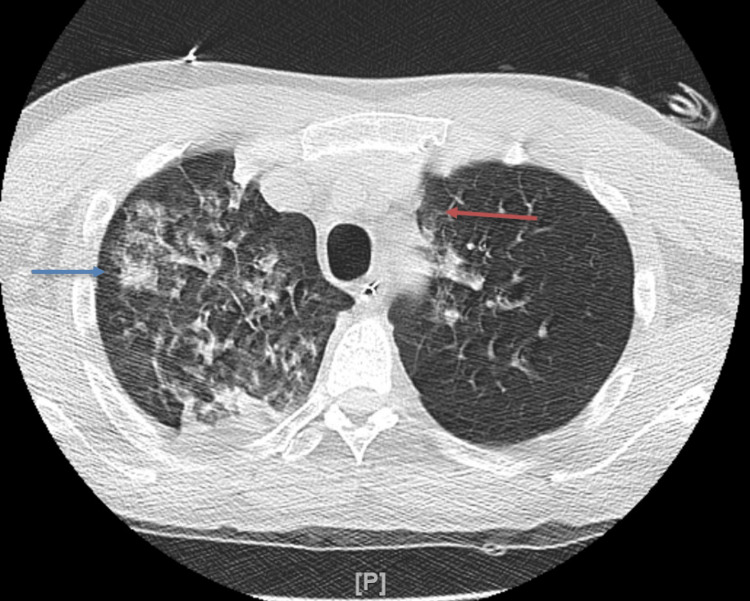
CT of the chest CT Chest revealed extensive pulmonary parenchymal findings detailed above including consolidation of the right lower lobe (blue arrow) which was considered a combination of both atelectasis and infection. Mediastinal lymphadenopathy (red arrow) was noted in addition to axillary lymphadenopathy
